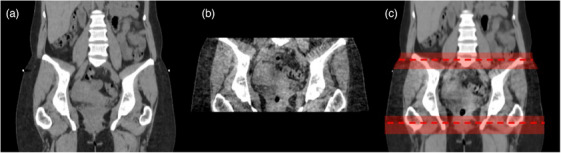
(a) pCT, (b) week 1 CBCT, and (c) mCBCT1. The edges of the original CBCT FOV are shown by the red dashed line. The rectangular regions are used for the local deformable image registration between indicate the areas used by the deformable image registration to stitch the pCT to CBCT to generate the mCBCT.
